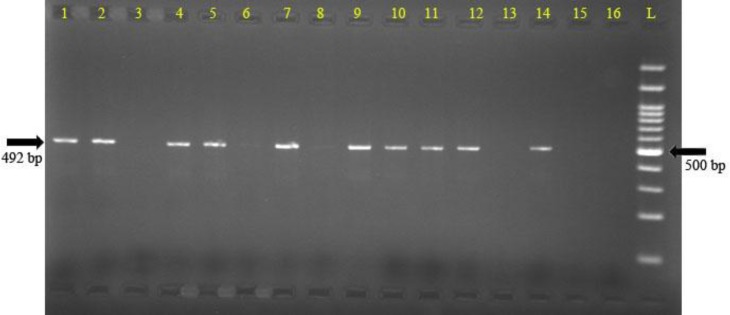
Genotyping of Xmn-1 polymorphism in patients with thalassemia intermediate. The picture demonstrates the results for 7 patients (two well for each patient, the first well for C and the second for T allele). Lanes (1, 2), (3, 4), (5, 6) are controls genotyped as CT, TT and CC respectively. Case 4 (lanes 7, 8) shows the TT genotype, cases 5and 6 (lanes 9 to 12) represent the CT genotype and case 7 (lanes 13, 14) reveals the CC genotype. Lanes 15 and 16 are negative controls. The 100 base pair (bp) ladder line has been depicted by “L”.
